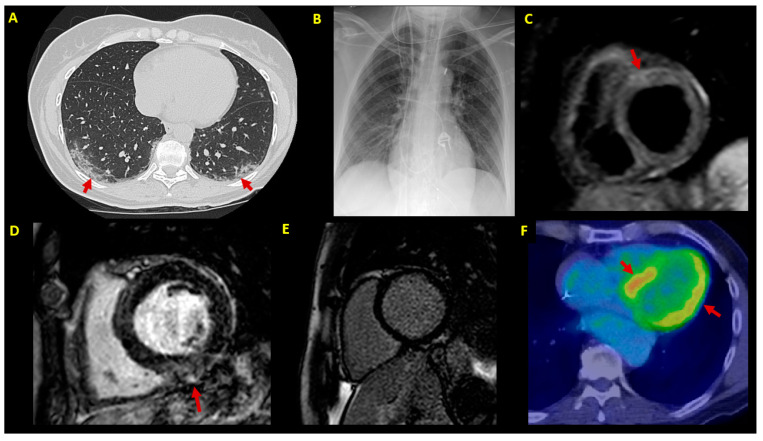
Imaging findings. Imaging findings at patient (P1–P7) diagnostic workup. (A) Chest CT scan showing bilateral patchy ground-glass opacities (arrows) (P3); (B) chest X-ray in a patient (P7) with cardiogenic shock supported by IABP, VA-ECMO and temporary pacemaker; (C) CMR in a patient with infarct-like acute myocarditis associated with COVID-19 (P3); T2-STIR sequence shows edema in the anterior basal segment (arrow); (D) LGE sequences in a patient (P5) showing mild inferior mid-myocardial/subepicardial LGE (arrow); (E) absence of LGE by 3-month follow-up CMR in a patient (P4) with fulminant myocarditis at presentation; (F) 3-month follow-up FDG-PET scan in an ICD carrier (P5) with virus-negative myocarditis; abnormal left ventricular FDG uptake is shown (arrows). CMR = cardiac magnetic resonance; CT = computed tomography; EMB = endomyocardial biopsy; FDG-PET= 18F-fluorodeoxyglucose positron emission tomography; IABP = intra-aortic balloon pump; ICD = implantable cardioverter defibrillator; LGE = late gadolinium enhancement; LVEF = left ventricular ejection fraction; STIR = short-tau inversion recovery; VA-ECMO = venoarterial extracorporeal membrane oxygenator.
